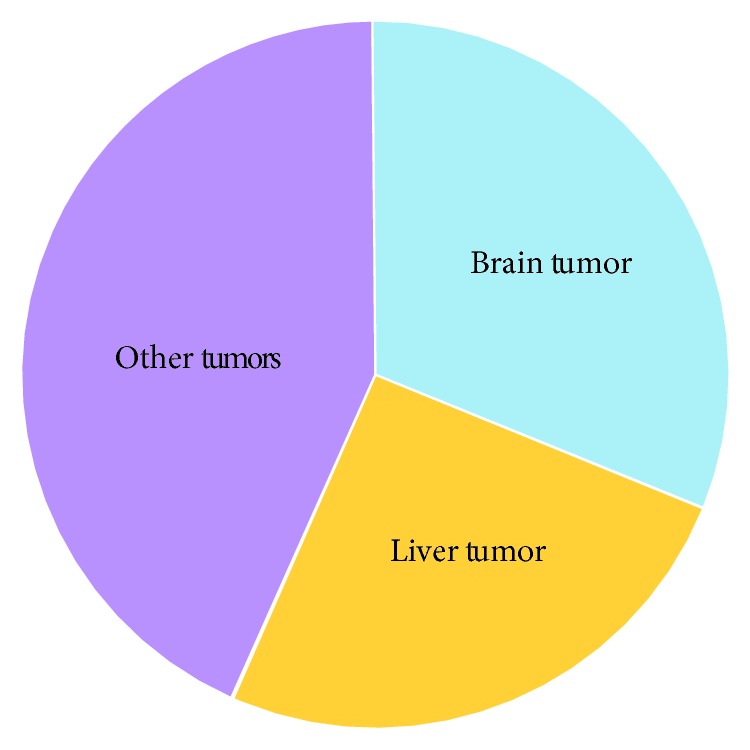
Summary review of the application of microbubbles combined with ultrasound for tumor therapy.
